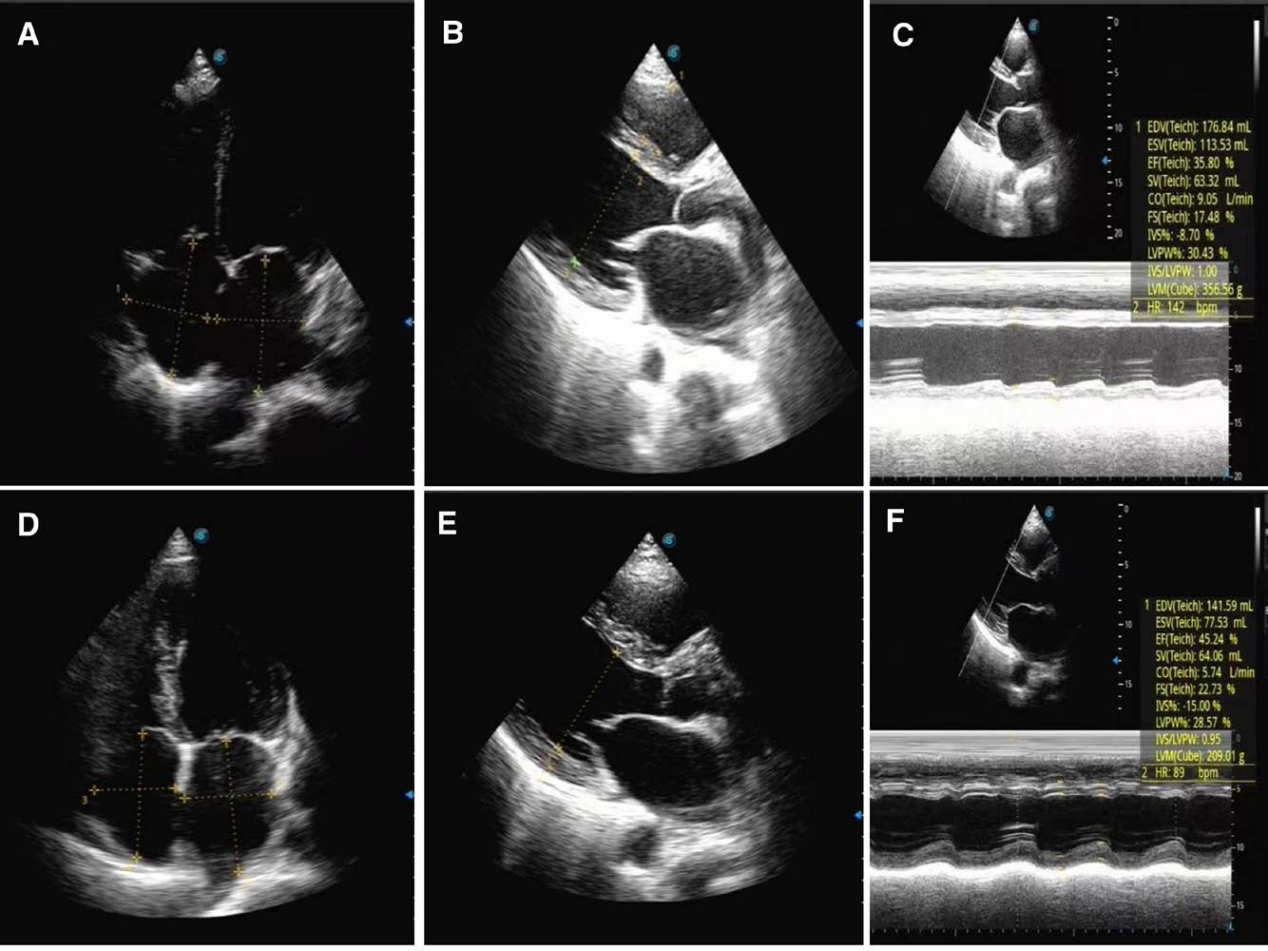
Echocardiograms from the first visit to the last follow-up. (A) Apical 4-chamber view at first visit; (B) Left ventricular long-axis view at first visit; (C) Indices of echocardiogram at the first visit. (D) Apical 4-chamber view at 1-mo follow-up. (E) Left ventricular long-axis view at 1-mo follow-up. (F) Indices of echocardiogram at 1-mo follow-up.
